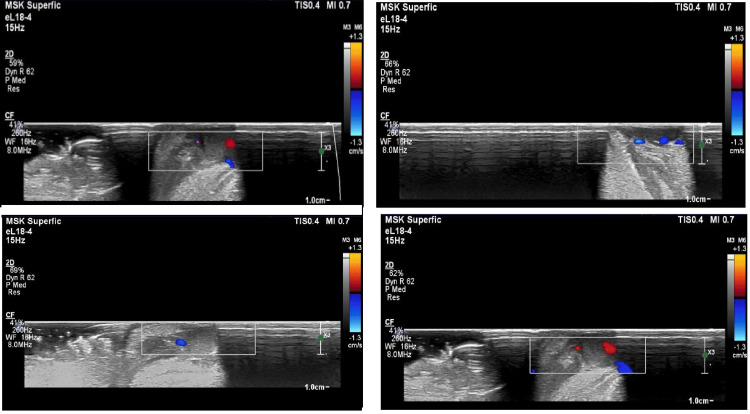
Ultrasound scan images of the case The squared boxes area shows the sluggish flow of blood
